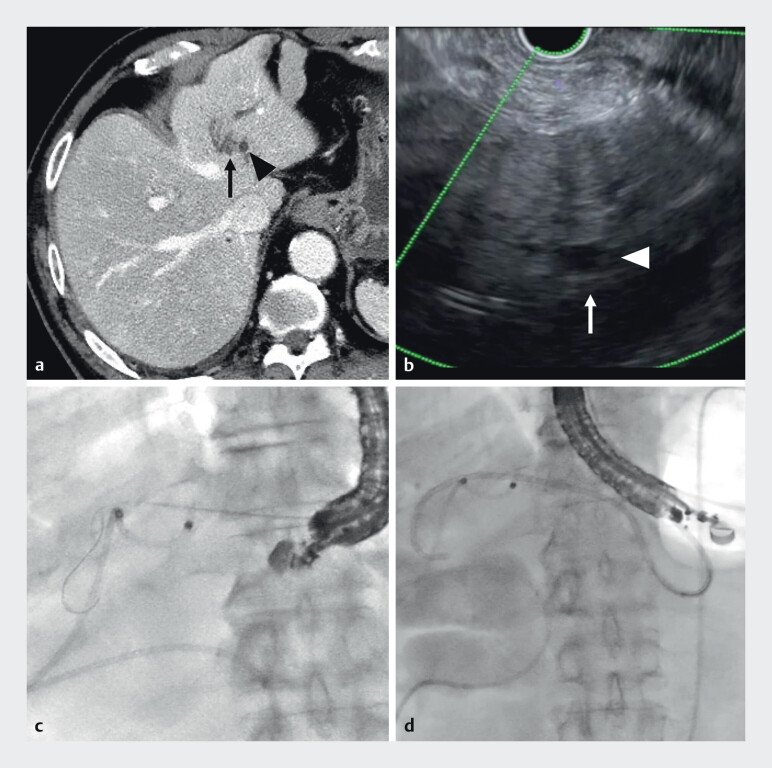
Endoscopic ultrasonography-guided hepaticogastrostomy.
